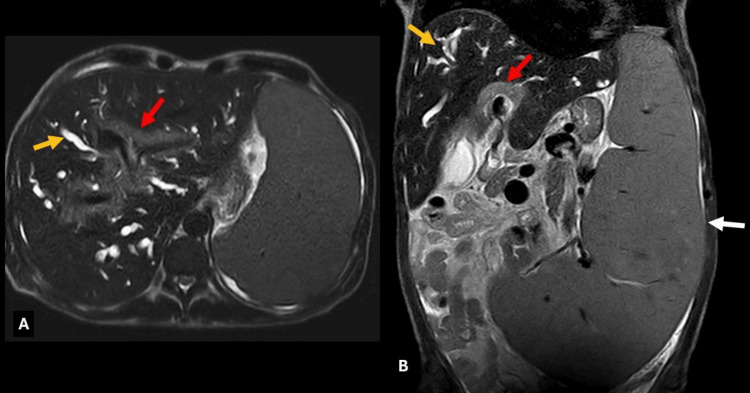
Contrast-enhanced MRI, T2 sequence Axial and coronal acquisitions showing slightly hyperintense periportal soft tissue without thrombosis (red arrows in A and B). There is dilation of the intrahepatic bile duct (yellow arrow in A and B). The coronal sequence shows massive splenomegaly (white arrow in B).
